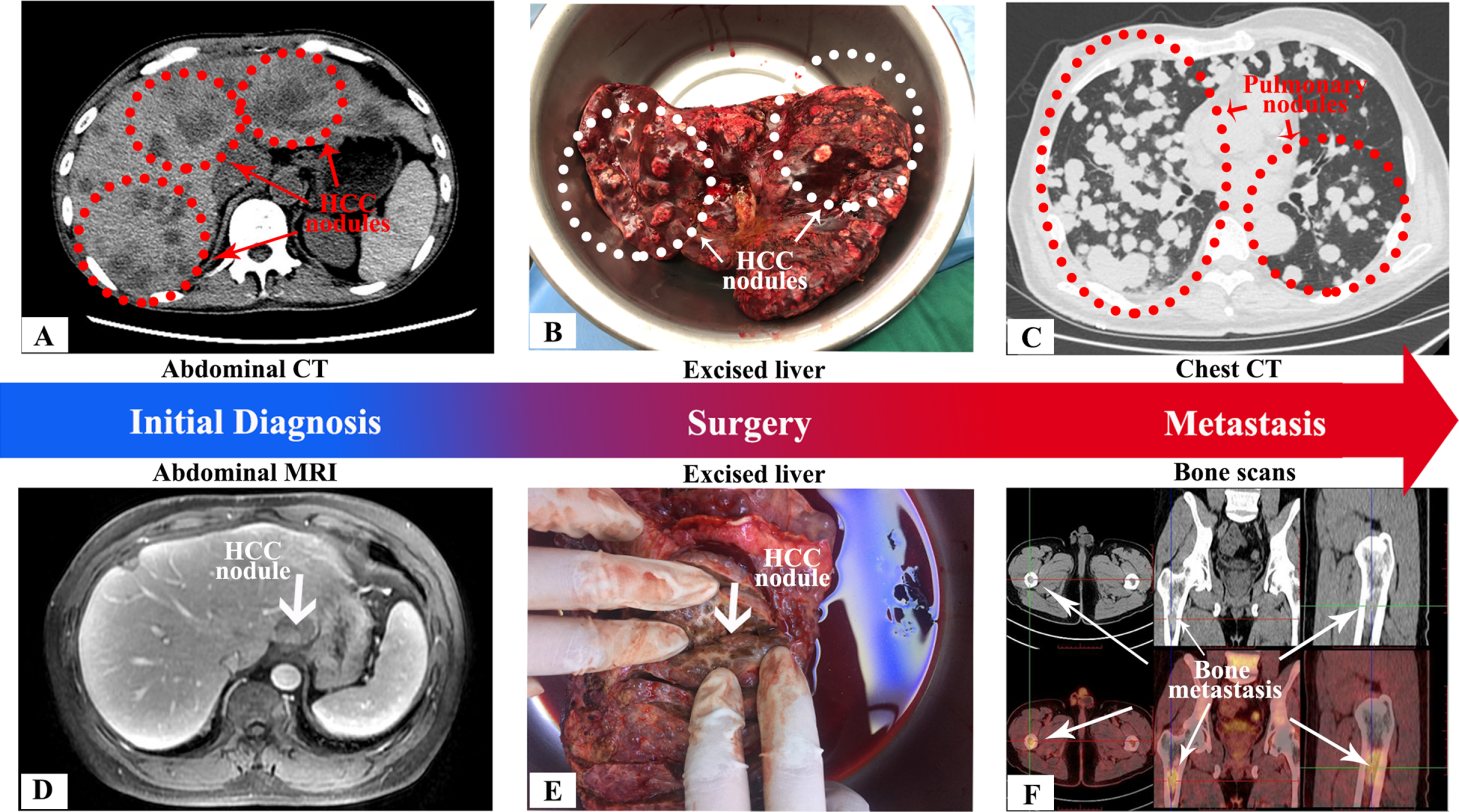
Preoperative CT and MRI scans, intraoperative tumor images and evidence of postoperative metastasis for two patients with tumor metastases. (A), (B), and (C) showed HCC patient no. 1. (A) Axial portal venous phase CT showed multiple, ill-defined lesions in liver (red line). The multiple lesions were presented hypodense, relative to the surrounding liver, in portal venous phase; (B) HCC nodules removed during surgery (white line); (C) Axial chest CT showed multiple, ill-defined, oval nodules in lungs (red line). The nodules were distributed at random. Combined with the clinical history, the lesions were considered as metastasis. (D), (E), and (F) showed HCC patient no. 2. (D) Axial arterial phase TIWI C^+^ showed well-defined, focal lesion in liver (arrow). The lesion was presented heterogeneous hypointense enhancement in later arterial phase; (E) HCC nodules removed during surgery (arrow); (F) Bone scans showed a focal lesion with hypermetabolism in right femoral cavity (arrow). There was no obvious bone destruction around the lesion. Based on the clinical history, the lesion was considered as metastasis. Photo credit: Xuebin Wang.
